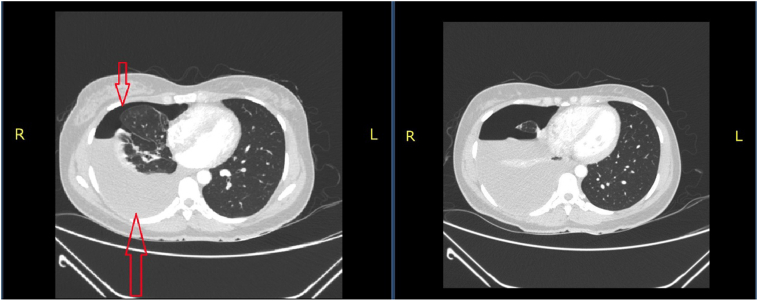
CT scan of the chest axial views - pneumohemothorax indicated by red arrows. (For interpretation of the references to colour in this figure legend, the reader is referred to the web version of this article.)
